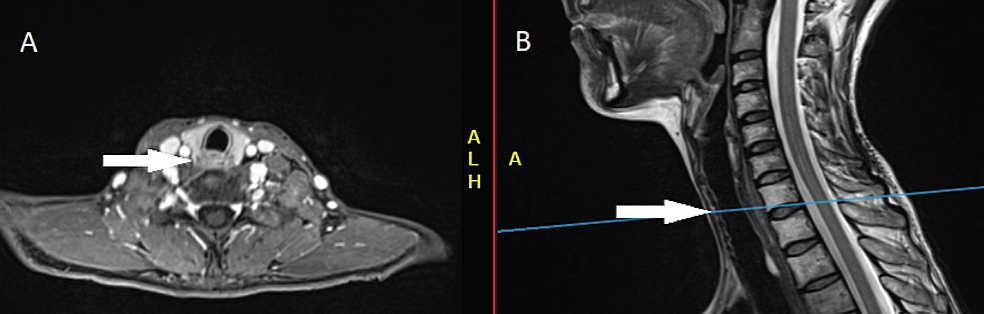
Follow-up imaging performed 24 months after completing the chemoradiation Axial (A) and sagittal (B) T1-weighted MRI still show a complete response to the treatment.
